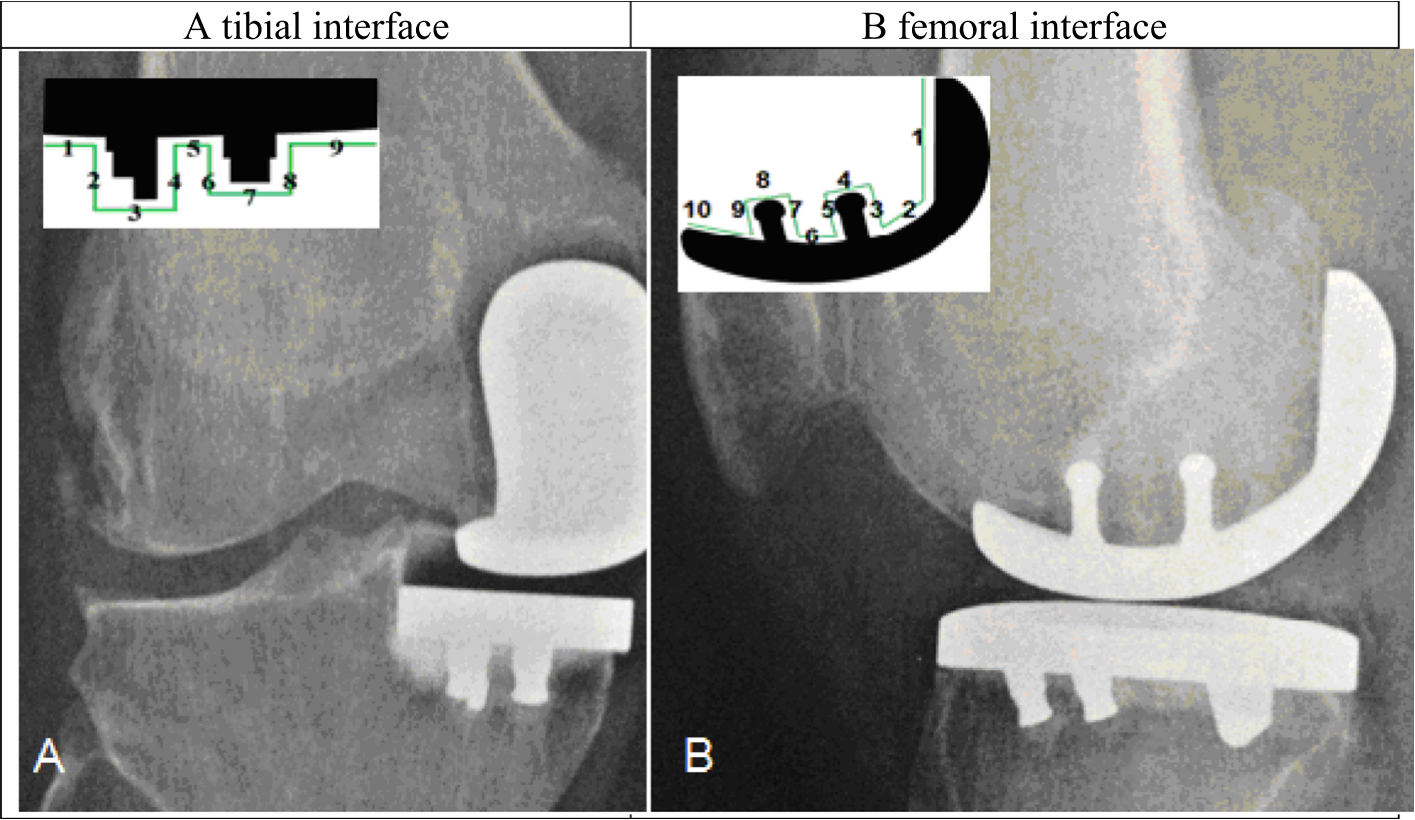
Radiographs with design of the specified regions for evaluation of presence and extent of radiolucency under the components
